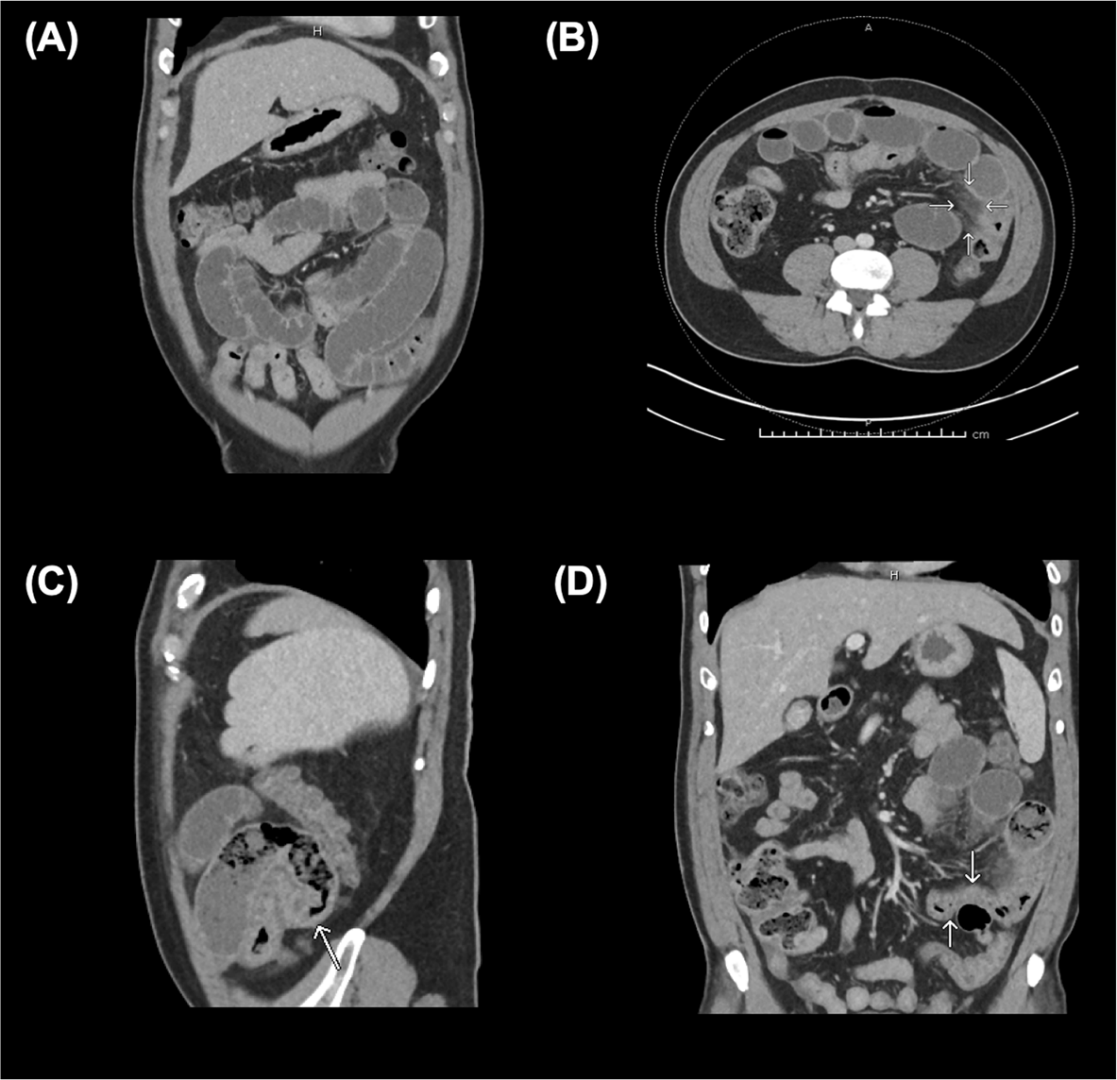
CT abdomen/pelvis with contrast. The CT scan of this CF patient demonstrated dilated loops of bowel proximally (a), with associated mesenteric venous congestion (b). Additionally, a transition point (c) was noted in the left hemiabdomen, distal to which the small bowel was decompressed (d). Just proximal to the transition point, gas bubbles and particulate matter were noted, suggestive of fecal contents in the small bowel
